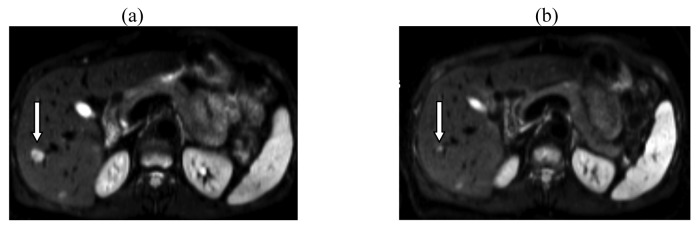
Diffusion-weighted MRI of the liver showing two metastases in the right lobe in (a) June 2009, and (b) February 2010. One metastasis (arrow) decreased from 15 mm in diameter to 7 mm, while the other remained unchanged (courtesy of Prof. Dr. E. Rummeny, Klinikum Rechts der Isar, Technische Universität München, Technical University of Munich, Germany).
